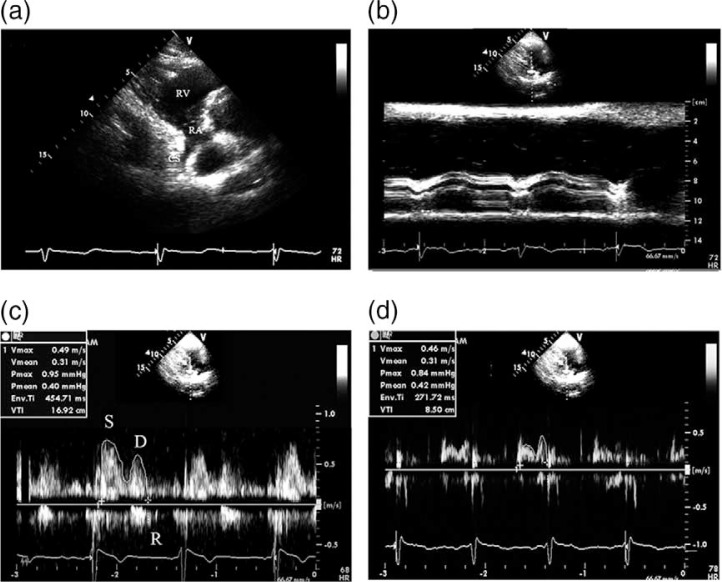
The measurement of coronary blood flow in the CS. (a) The CS seen from the parasternal right ventricular inflow tract view. (b) The measurement of the diameter of CS using adjust M-Mode ultrasonography. (c) The Doppler spectrum of coronary blood flow in the CS obtained from the parasternal right ventricular inflow tract view and the measurement of Doppler parameters of coronary blood flow in the CS by digitized Doppler spectral envelopes in normal subjects. (d) The measurement of Doppler parameters of coronary blood flow in the CS by digitized Doppler spectral envelopes in patients with CAD (d). RA = right atrium; RV = right ventricle; CS = coronary sinus; S = systolic wave of the antegrade blood flow; D = diastolic wave of the antegrade blood flow; R = retrograde blood flow; Env.Ti = duration of measured envelope; CAD = coronary artery disease.
